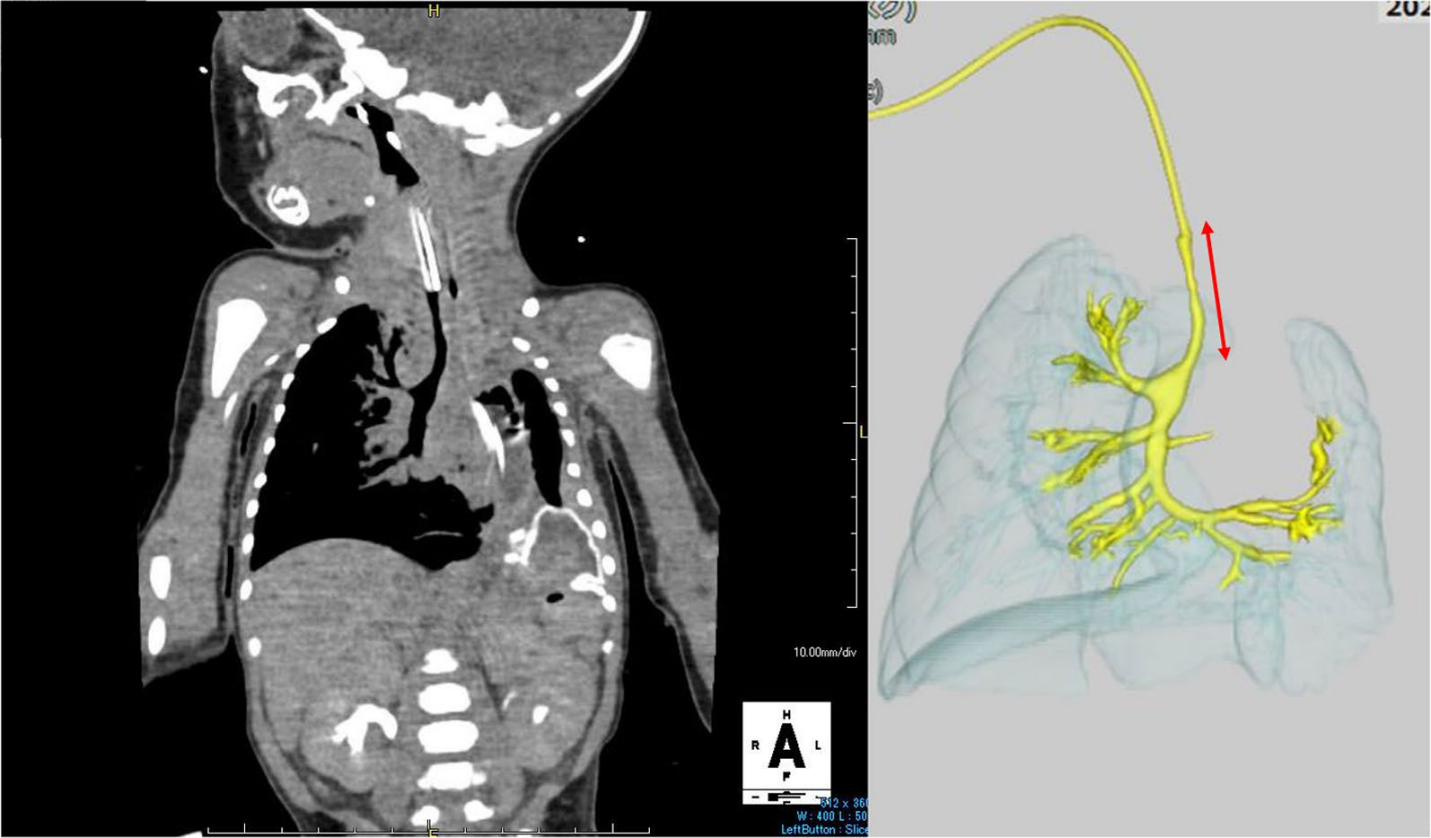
Enhanced CT confirmed the diagnosis of CTS (arrow), and left lung agenesis. The tracheal stenosis was approximately 4 cm in length, extending from upper trachea to the just above right upper lobe bronchus
